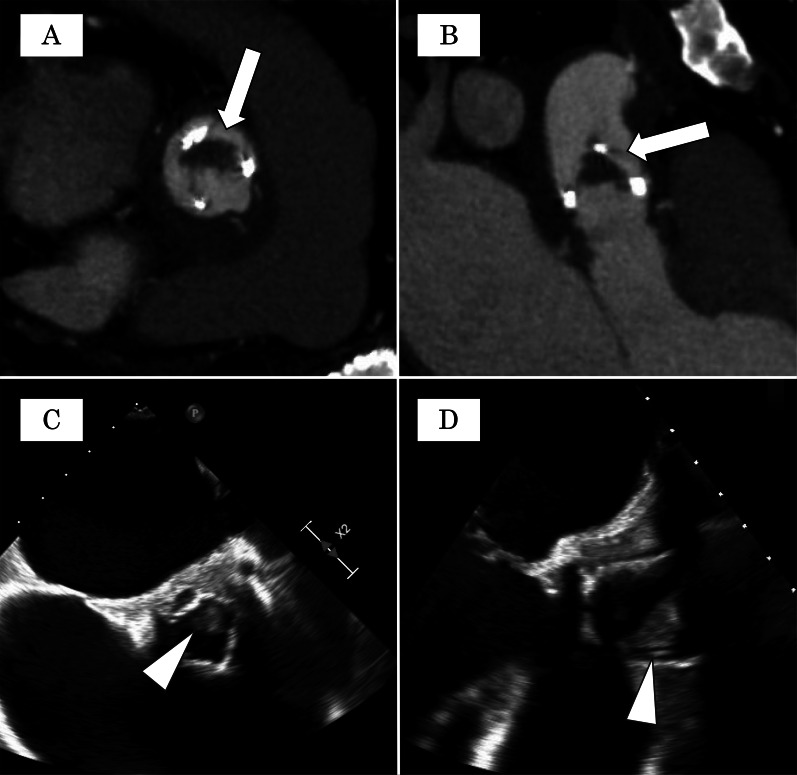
Preoperative echocardiography. The white arrow points to a low-density mass on the leaflet by CT scan, suggesting vegetation (A short axis view, B long axis view). The arrowhead indicates a low echoic and mobile mass by transthoracic echocardiography (C short axis view, D long axis view)
